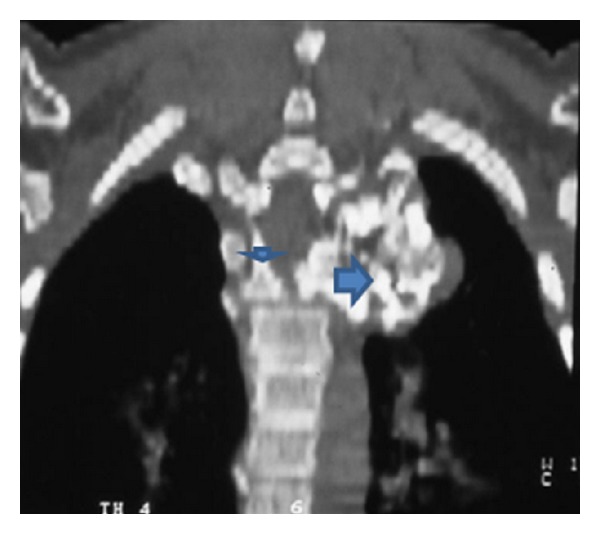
Coronal reformatted CT scan image showed a huge intraspinal mass with heterogeneous intensity. The ossified matrix is surrounded by cartilage tissue. The osseous tumour originating from the left T3–5 facet joint (arrows-arrow head is the pedicle and long arrow is the exostosis).
